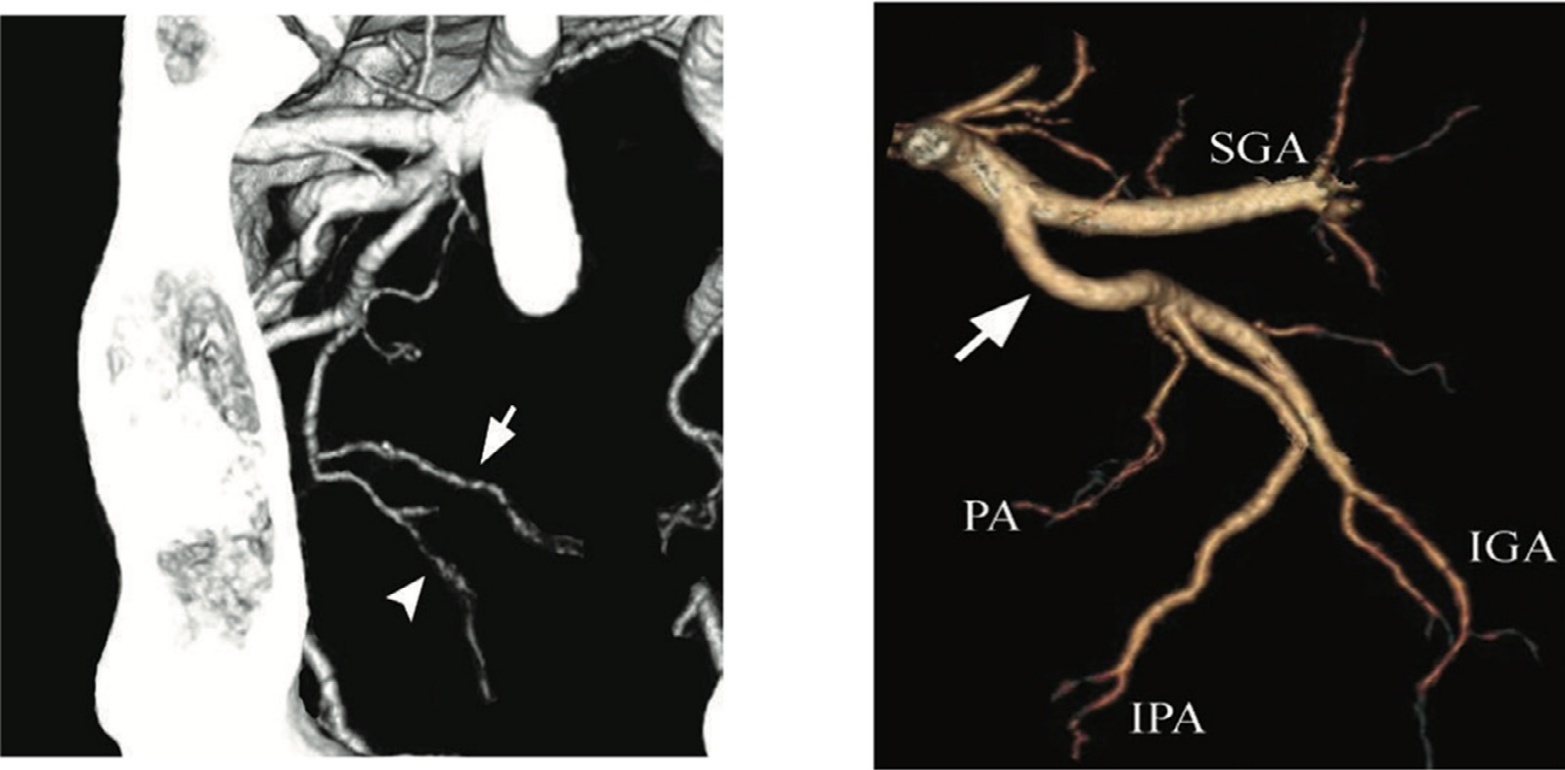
MIP and 3D pelvic CTA of the prostate artery branching pattern (a, b-) ([18],[28])
